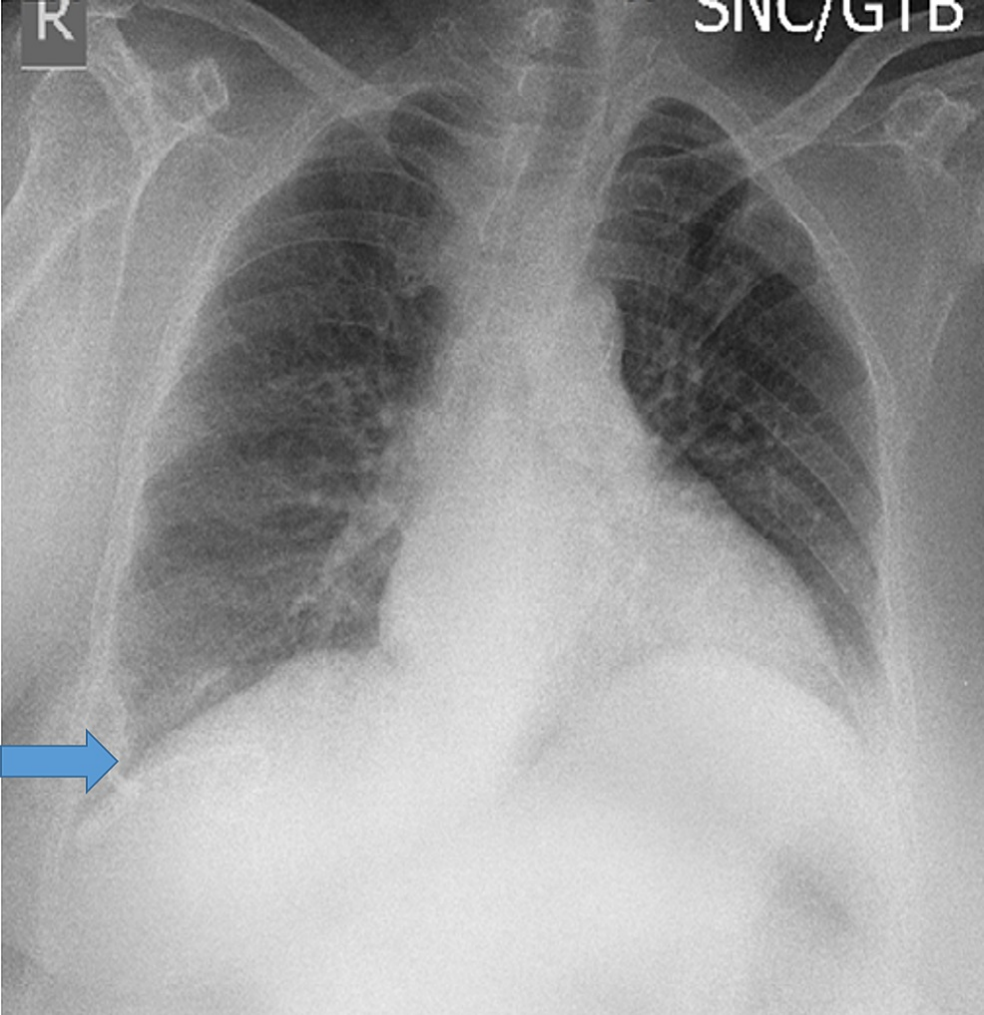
Chest radiograph showing complete lung expansion and drainage of pleural fluid
